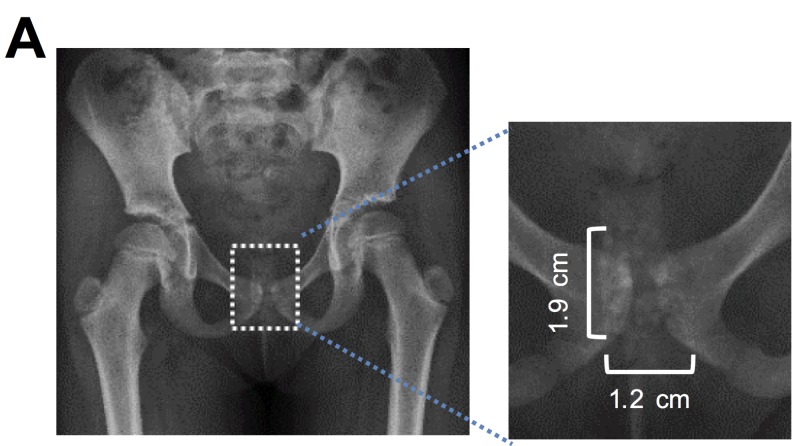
Pelvic X-ray showing a cluster of rounded calcific densities projecting over the symphysis pubis
